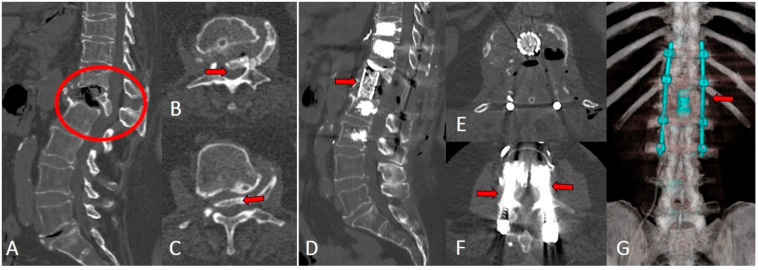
Representative imaging of a patient with an unstable OF5 fracture before (A–C) and after surgery (D–G). (A) Sagittal CT reconstruction showing a comminuted vertebral fracture with compression of the spinal canal (red circle). (B,C) Axial CT images demonstrating displaced bone fragments within the canal (arrows). (D) Sagittal CT reconstruction after placement of a titanium mesh cage (arrow) filled with autologous bone, showing restoration of vertebral height and formation of spondylodesis. (E) Axial CT view of the interbody cage positioned centrally. (F) Axial CT image showing bilateral transpedicular screw placement (arrows). (G) Three-dimensional CT reconstruction demonstrating correction of thoracolumbar alignment and coronal balance after cage insertion and posterior instrumentation.
